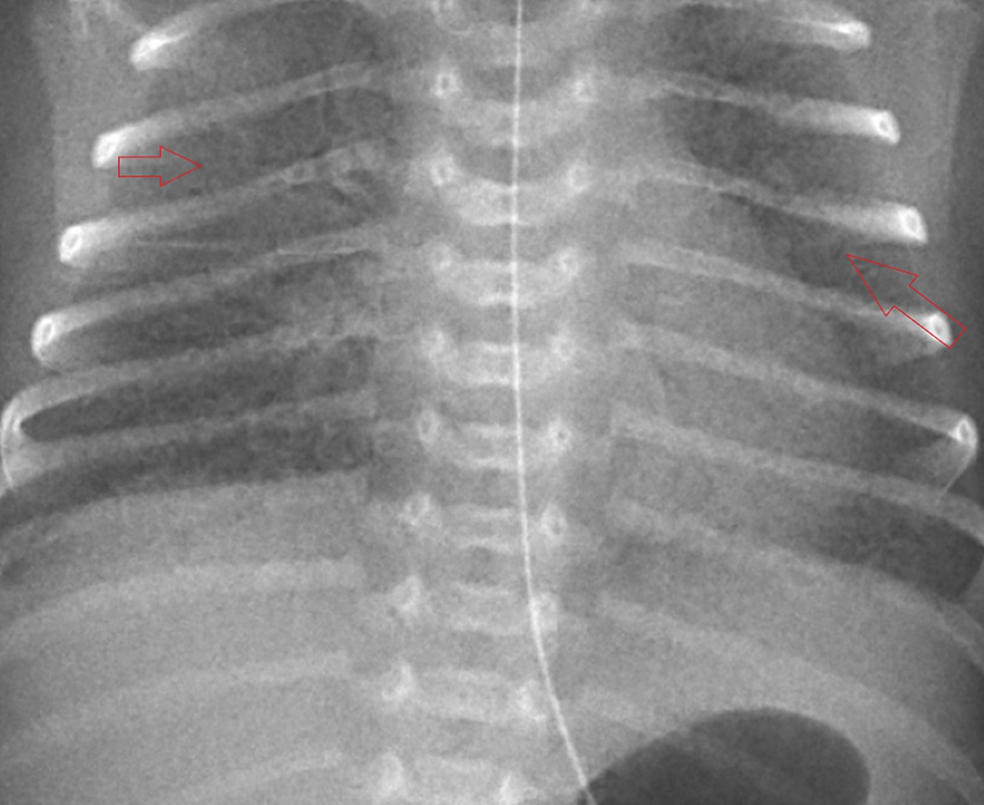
Chest x-ray taken immediately after birth indicate bilateral interstitial infiltrates (arrows)
